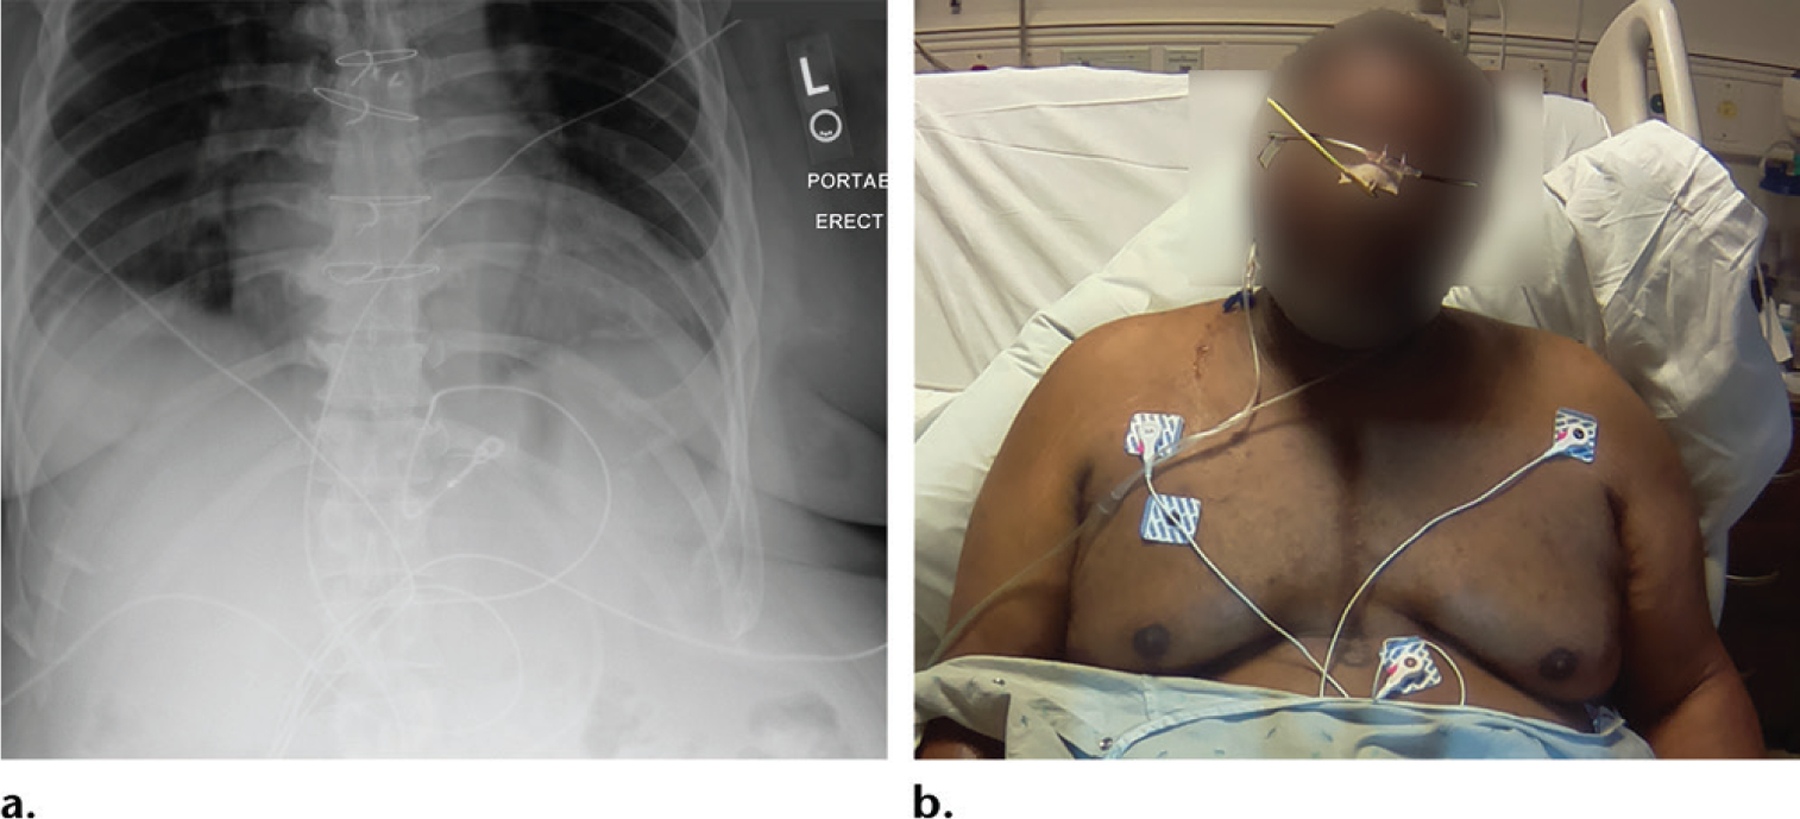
Thoracoabdominal radiograph obtained to evaluate for feeding tube placement. (a) Radiograph does not show a feeding tube, a finding that would have prompted the radiologist to call the floor nurse to ascertain if a tube was in place. (b) Photograph shows the patient with the feeding tube, which has possibly coiled in the upper chest or neck. This requires an urgent and confident call from the radiologist to reposition the tube.
